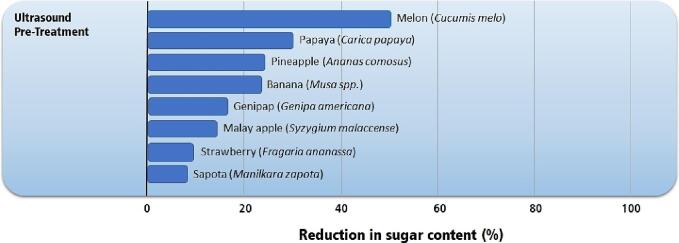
Reduction in sugar content in fruits by applying ultrasound pretreatment [81], [83], [84], [89], [90], [91], [107].
